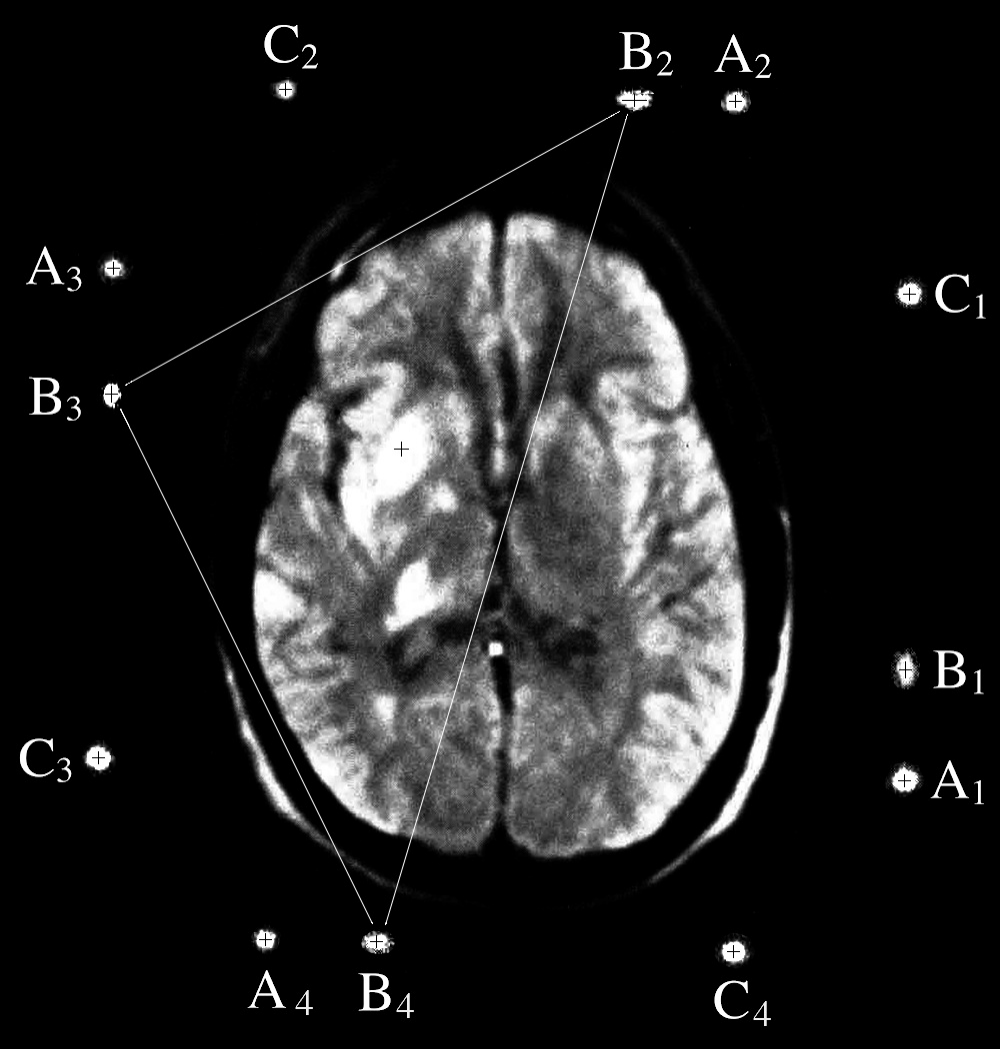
MR Image with Four Sets of Fiducials MR image of a patient to whom a first-generation BRW MR localizer frame (Radionics Inc., Burlington, MA) is attached. Four N-localizers create four sets of fiducials \begin{document}\left\{ \mathrm{A_1,B_1,C_1} \right\}\end{document}, \begin{document}\left\{ \mathrm{A_2,B_2,C_2} \right\}\end{document}, \begin{document}\left\{ \mathrm{A_3,B_3,C_3} \right\}\end{document}, and \begin{document}\left\{ \mathrm{A_4,B_4,C_4} \right\}\end{document}. The black cross hairs indicate the centers of the fiducials and the target point \begin{document}P_T\end{document}. Adapted from [11].
